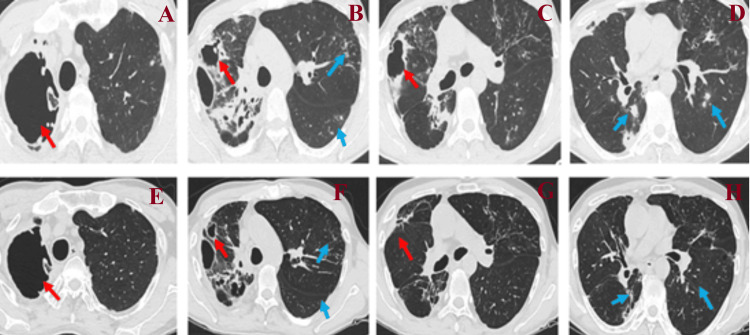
CT scan of patient 1 Figure panels A, B, C, and D present imaging before treatment, and panels E, F, G, and H present imaging at the end of the initial phase of treatment. A decrease in the size of the large cavities (red arrows) and a decrease in the extent of pulmonary infiltrates (tree-in-bud lesions) (blue arrows) are observed.
